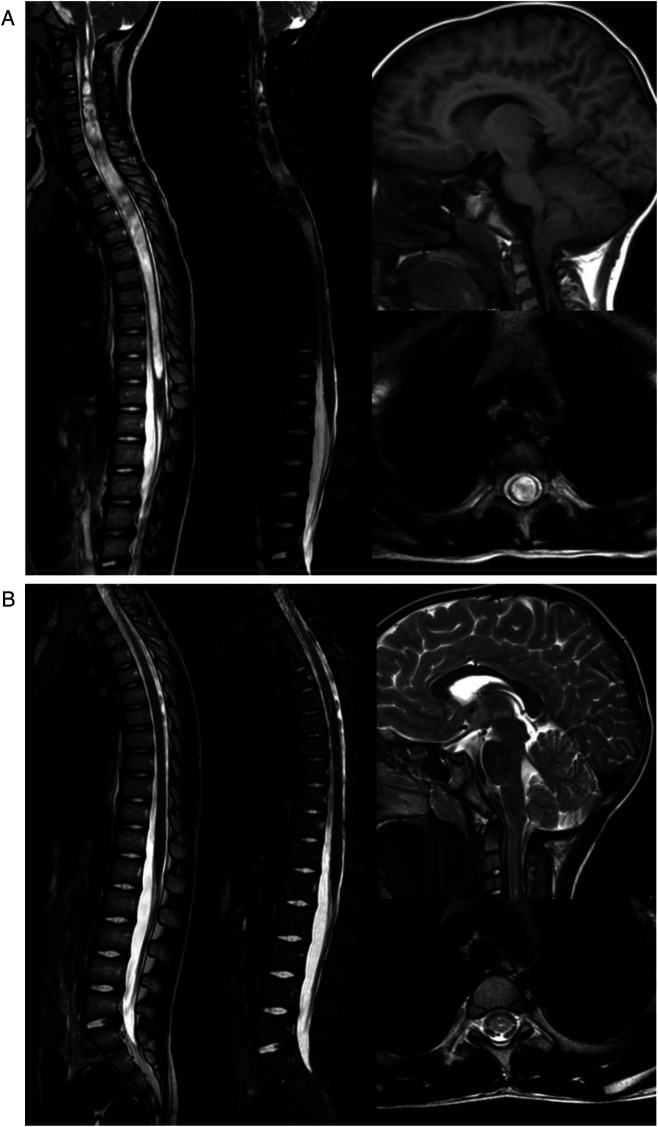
(A) This is a 4-year-old boy who presented with dizziness and occipital-cervical pain. The MRI results of the cephalic and spinal cord showed that the medulla oblongata and pons were compressed and deformed, the foramen magnum was crowded, and the inferior margin of the cerebellar tonsil exceeded the level of the foramen magnum, about 1.1 cm. Multiple beaded-like abnormal signals were visible in the spinal cord. The lesion extended up to the clivus and down to the inferior margin of the 11th thoracic vertebra. The diameter of the syringomyelia was about 1.0 cm, the length of the syringomyelia was 22.55 cm, and the volume of the syringomyelia was 17.7 cm^3^. The CSC-MO was 102°. (B) This was reviewed 2 years after operation. The MRI results of cephalic and spinal cord showed that the medulla oblongata and pons were normal, the cerebellar space was clear, and the lower edge of cerebellar tonsil was located above the foramen magnum. The multiple beaded signals in the spinal cord have disappeared, and the compression of the spinal cord parenchyma has decreased compared to pre-operative findings. The length and diameter of the syringomyelia after surgery cannot be measured. The CSC-MO was 155°.
